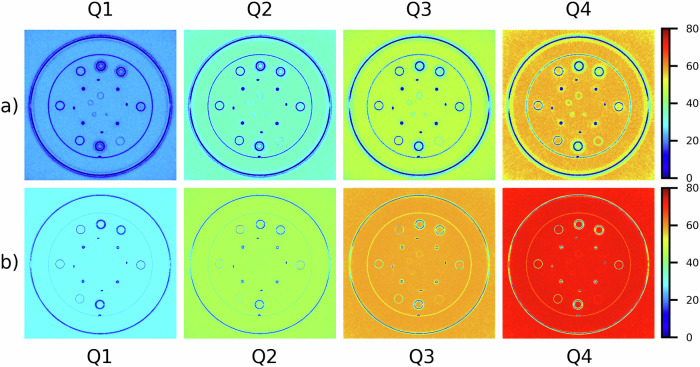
Photon-counting detector computed tomography scanner: for the CTP404 module, maps of the percentage difference (%) between noise of FBP-reconstructed image and noise of QIR-reconstructed images with different iterative powers (*i.e*., Q1, Q2, Q3, and Q4), using the smoother (a) and sharper (b) reconstruction kernel. FBP, Filtered back projection; QIR, Quantum iterative reconstruction
